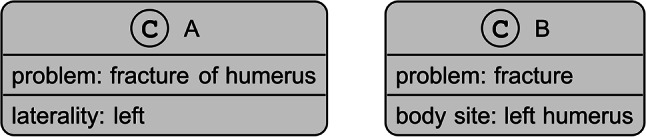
Two methods for combining structure and terminology. The same information is present in both A and B, and if they are both using SNOMED CT, it is possible to transform between the two structures
